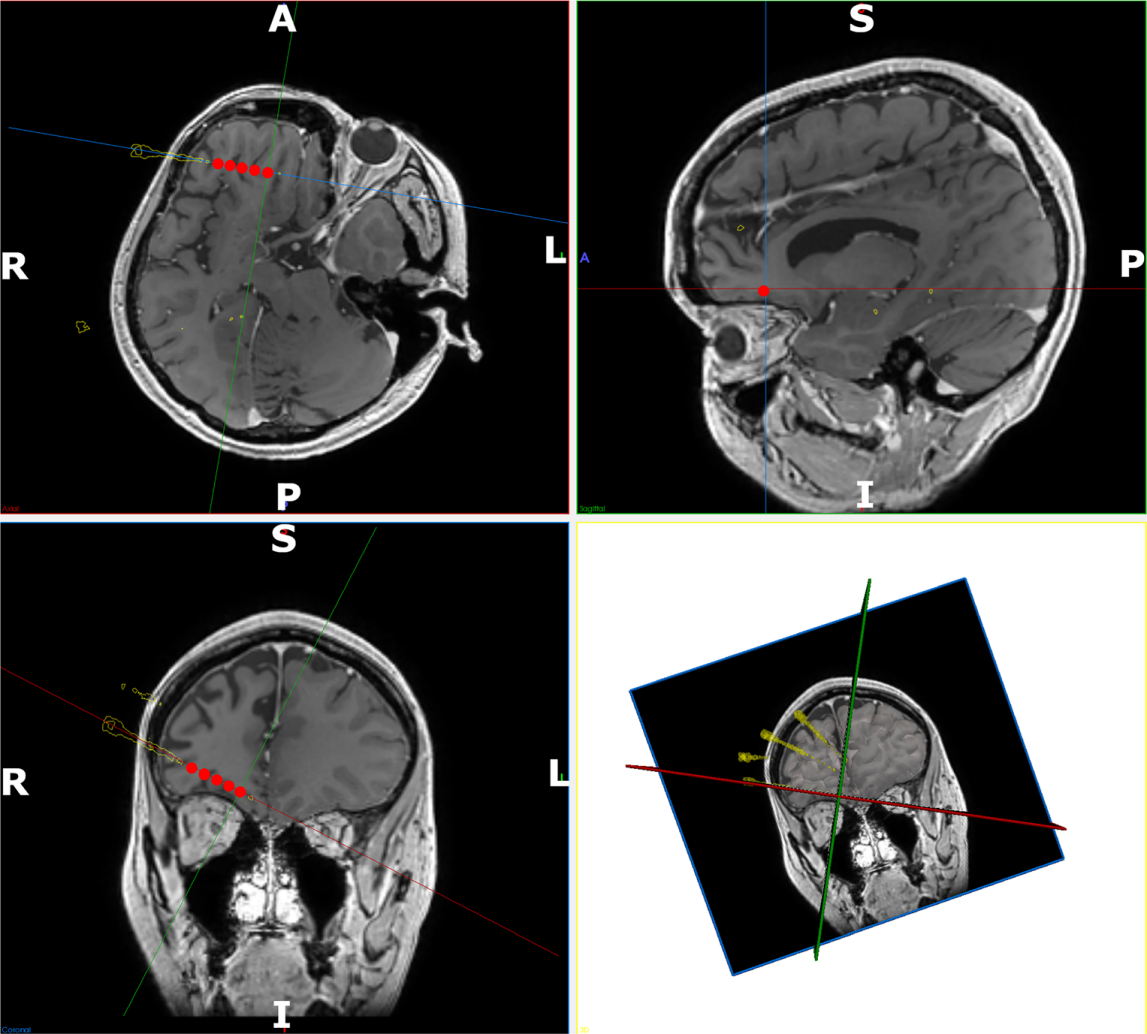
Detailed post-implantation view of active contacts: detailed views of the contacts that were active on the right orbitofrontal electrode at the onset of the seizure. Implemented electrode trajectories segmented from the post-operative CT are shown (yellow) and fused with the preoperative MRI. The electrode contacts active at the onset of the seizure are shown in red. These have been accentuated for clarity. In-line trajectory views (top left and bottom left) as well as probes eye view (top right) and 3D model (bottom right) are shown. Note: the orbitofrontal trajectory passes through the gray matter at the depths of the sulci along the orbitofrontal cortex before terminating in the mesial prefrontal cortex. Electrode conflicts with vessels in the sulcus are averted by preventing the trajectory from crossing sulcal pial boundaries
